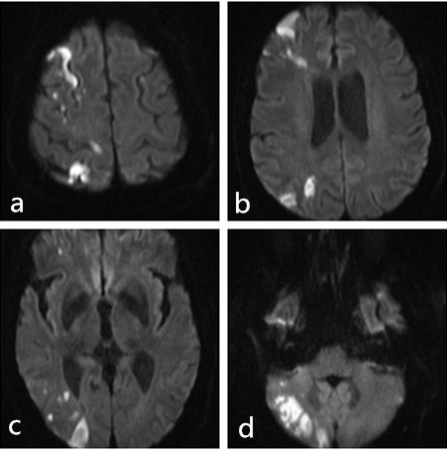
MRI showed multiple areas of infarct in the right side of the cerebrum and cerebellar hemisphere.
